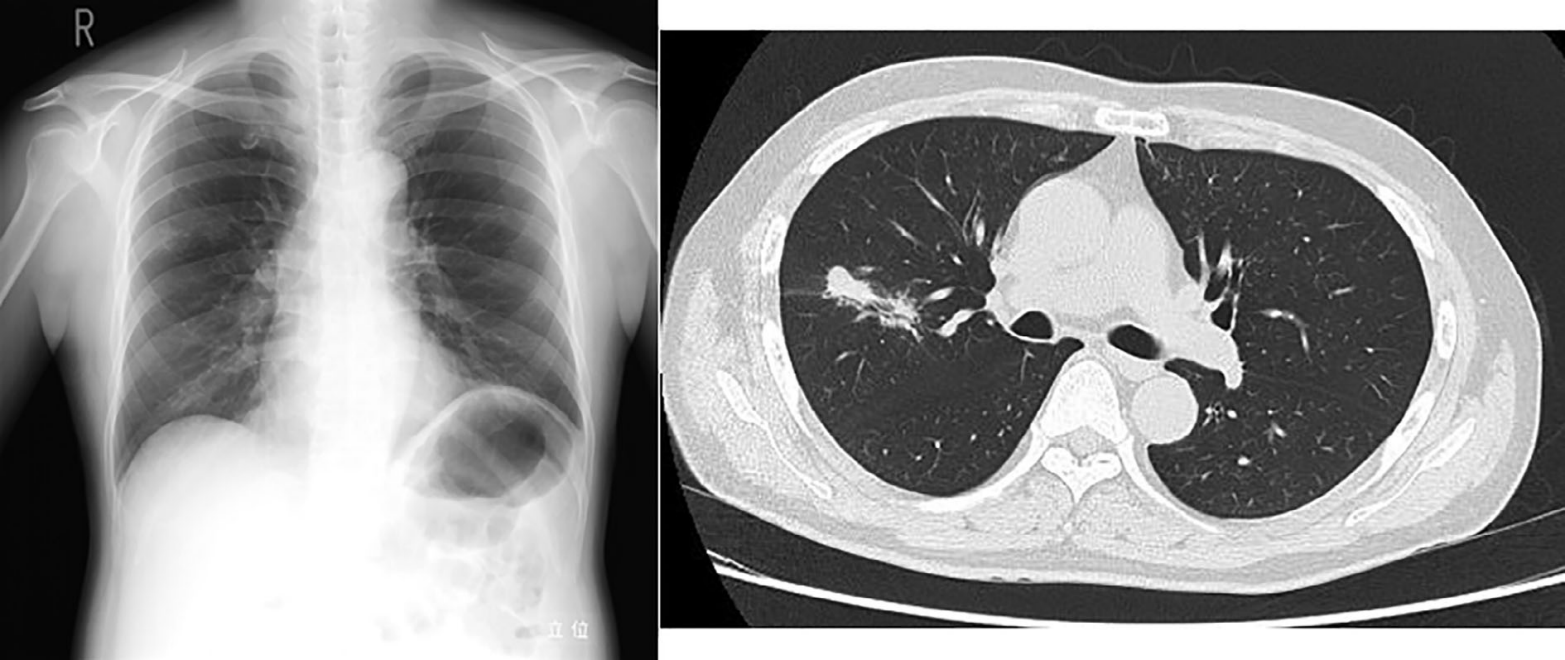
Chest X‐ray and computed tomography before operation. An abnormal infiltrative shadow is indicated in the right upper lung field on the chest X‐ray. A dumbbell‐shaped nodule measuring 40 mm × 10 mm is seen in the right upper lobe on the chest computed tomography.
